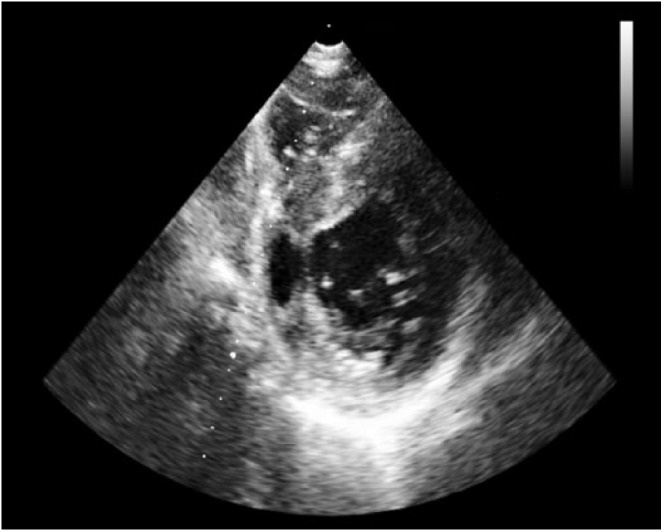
Parasternal short axis view of transthoracic echocardiogram across LV showing outpouching of inferoseptal and inferior wall.
